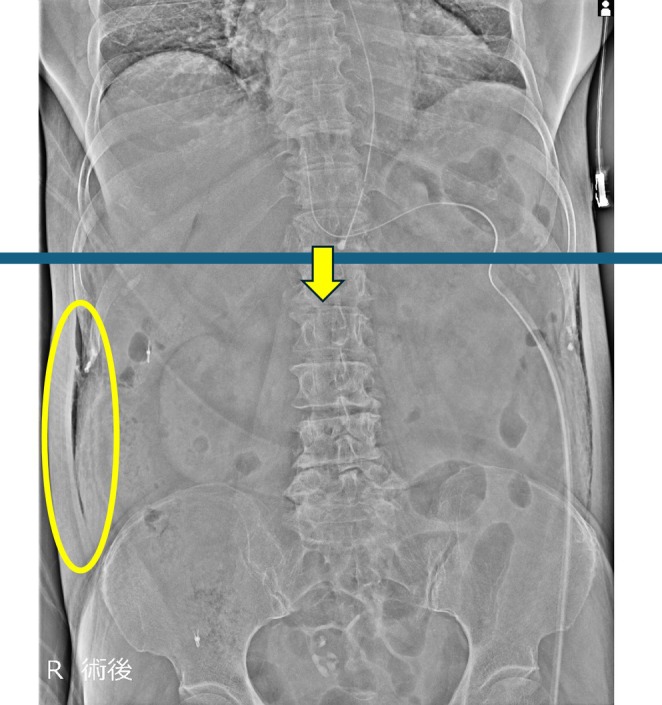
Representative postoperative abdominal radiograph of an SE‐negative case. Subcutaneous emphysema is confined to the abdominal wall at or below the level of the T12 thoracic vertebra (horizontal blue line). A small amount of gas is visible along the flank but does not extend cranially beyond T12.
